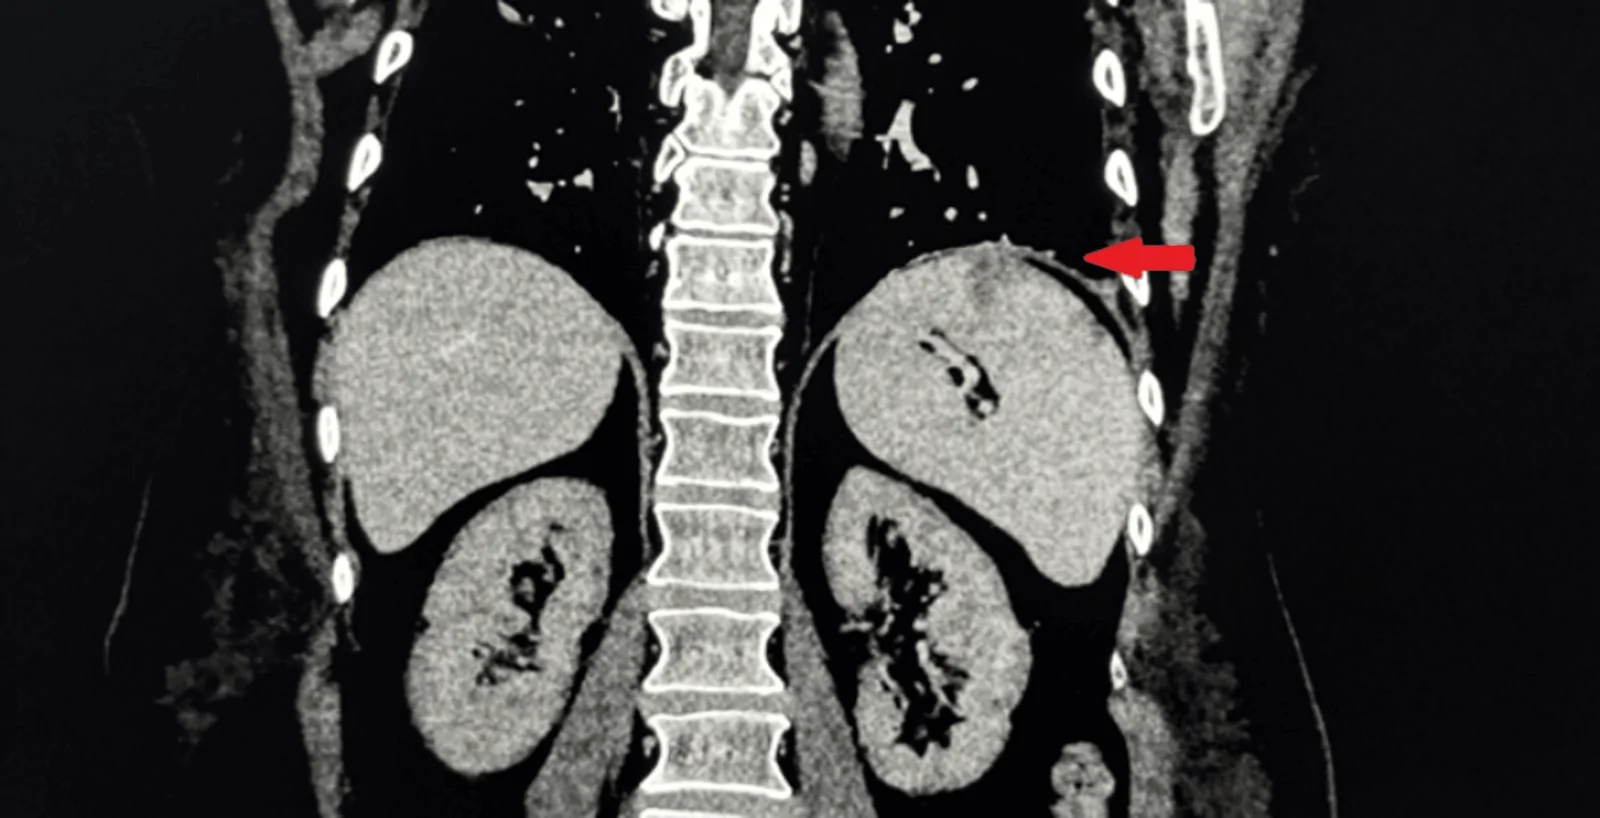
Coronal contrast-enhanced CT abdomen demonstrating a wedge-shaped hypodense region in the spleen indicating splenic infarct (red arrow), consistent with thrombotic involvement in catastrophic antiphospholipid syndrome (CAPS).
